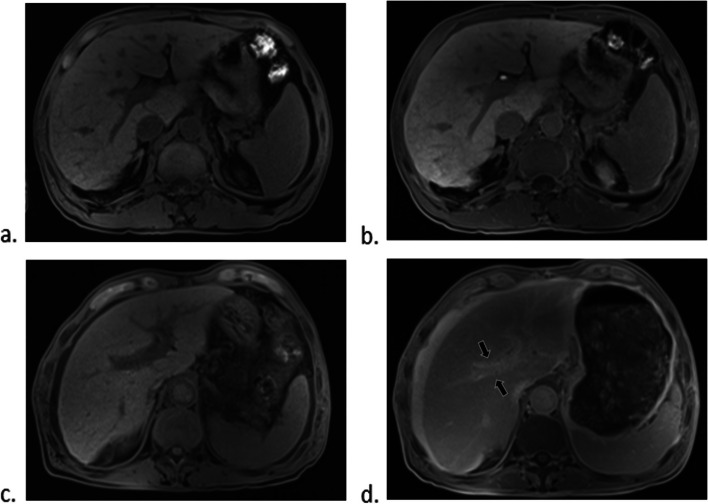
The pre-contrast T1-weighted image (a) and HBP image (b) were selected from a 57-year-old compensated cirrhotic patient. Periportal hyperintensity on HBP is not observed in this patient. The pre-contrast T1-weighted image (c) and HBP image (d) were selected from a 48-year-old decompensated cirrhotic patient. Periportal hyperintensity on HBP is observed (black arrow) in this patient. HBP, hepatobiliary phase
